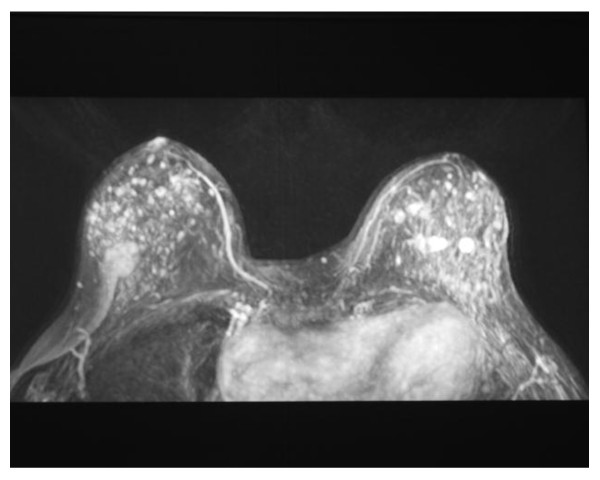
T1-weighted breast MRI. Representation of well-circumscribed, oval, multifocal, bilateral high signal intensity lesions.
